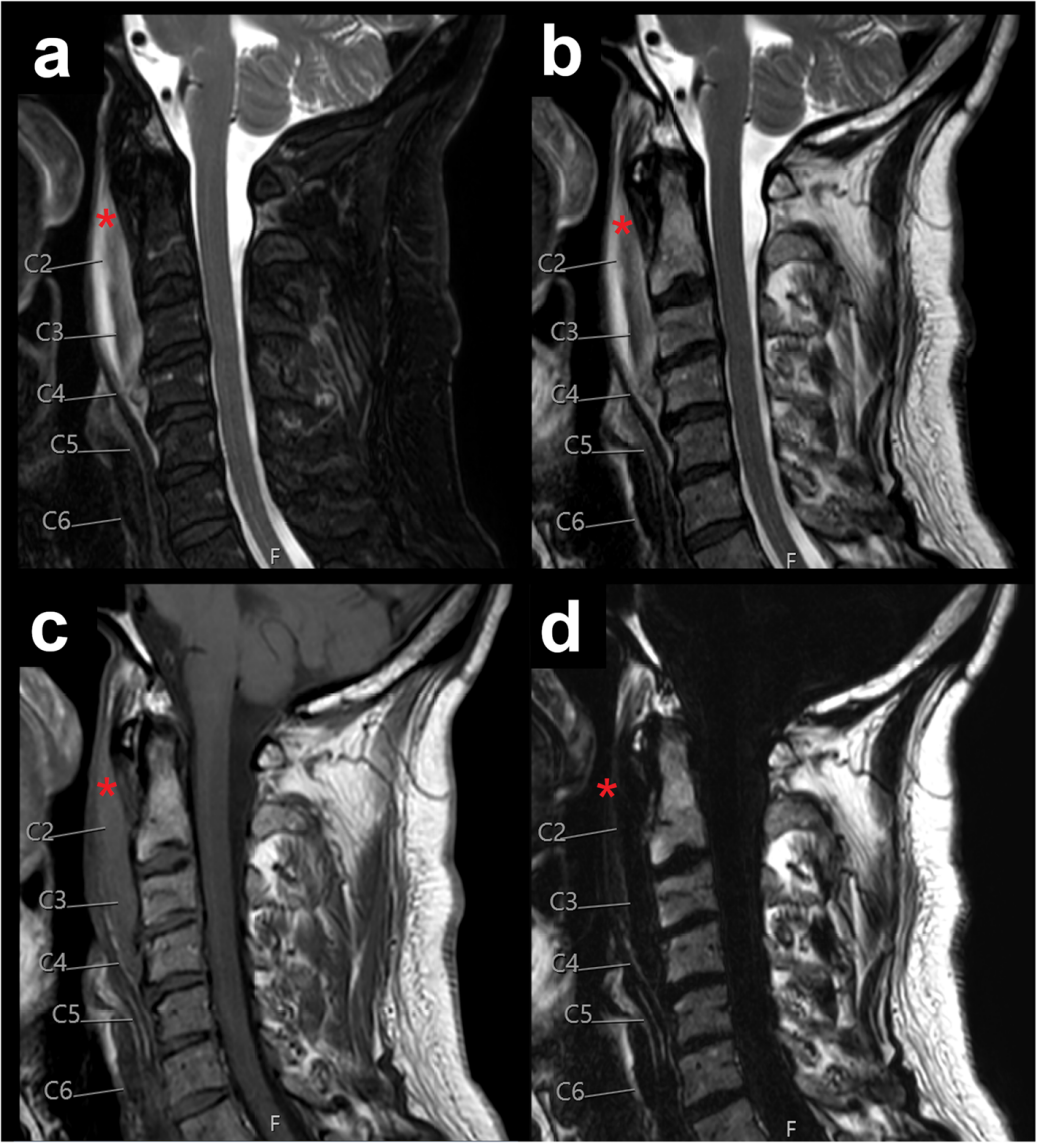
Traumatic cervical prevertebral haematoma. This figure shows the cervical spine of a 55-year-old male patient using water-only images (a), in-phase images (b), and fat-only images (d) of the sagittal T2-weighted DIXON sequence, together with a sagittal T1-weighted sequence (c). The patient had suffered a traffic accident and shows a cervical prevertebral haematoma spanning from C1 to C5 (red asterisks)
